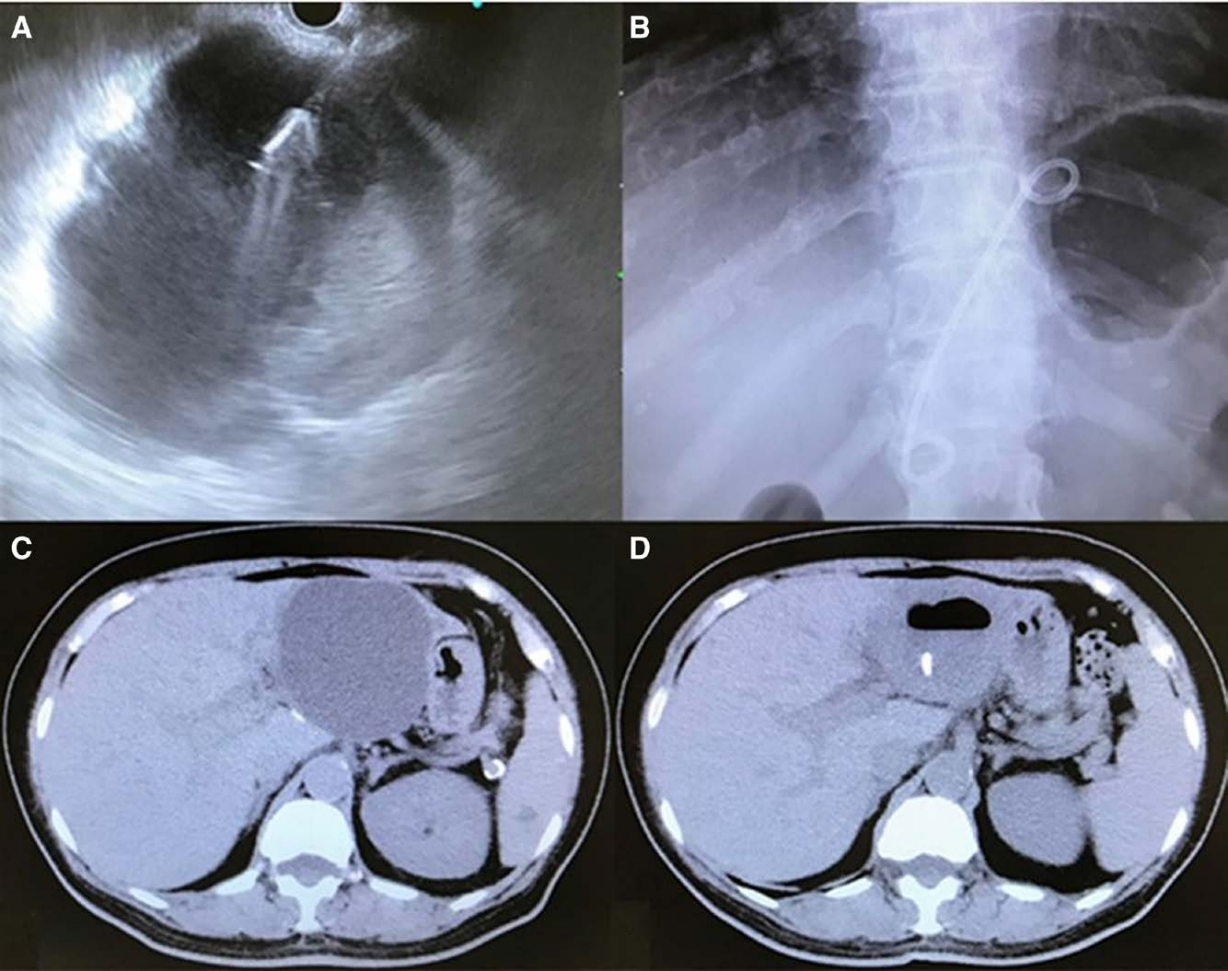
EUS-guided drainage of symptomatic SHC. (A) 19-gauge needle is inserted into the cavity. (B) A 7-Fr 10 cm DPPS is placed. (C) CT scan reveals a large cyst in left lobe of the liver. (D) CT scan confirms that the drainage tube is placed in the cavity of the hepatic cyst. CT = computed tomography, DPPS = double pigtail plastic stent, SHC = simple hepatic cysts.
